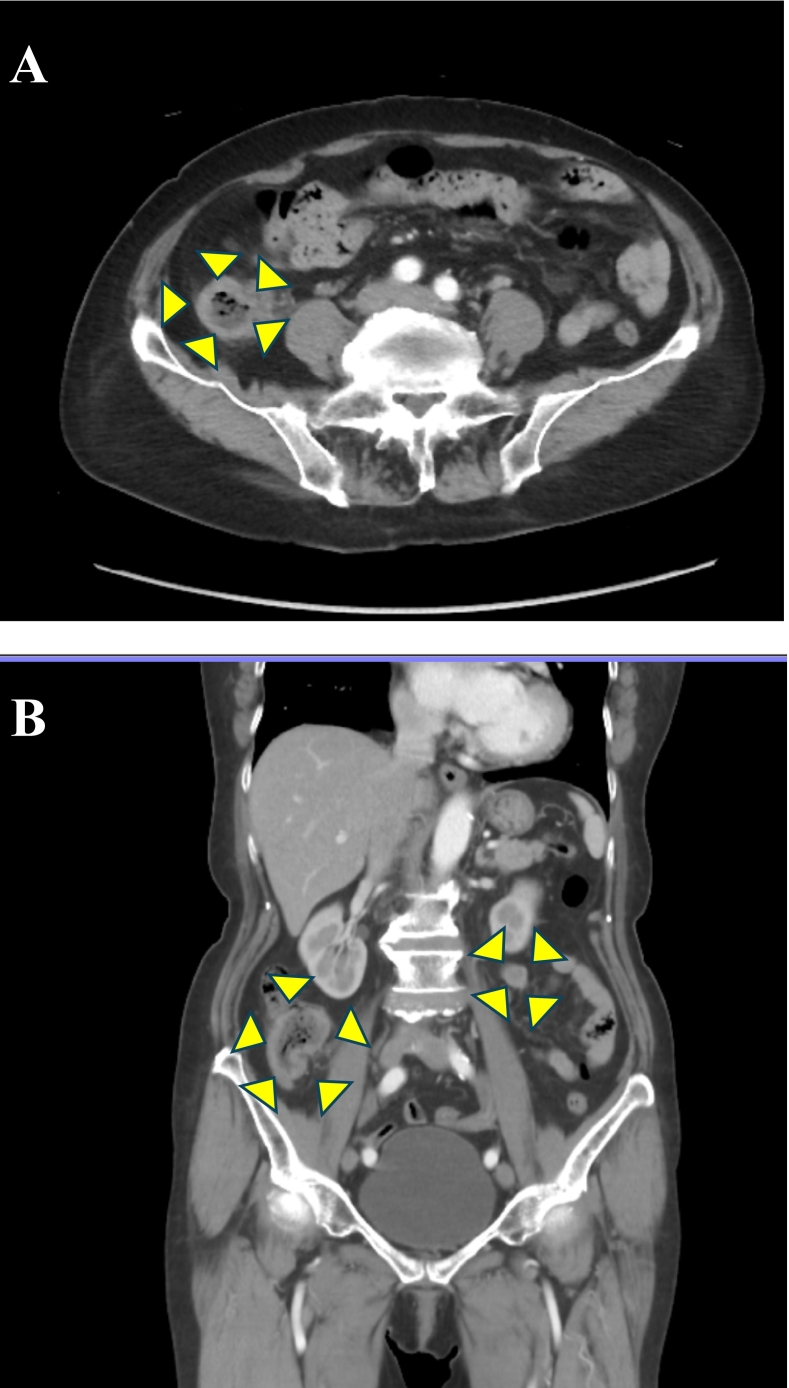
Abdominal computed tomography (CT). A and B: CT demonstrated an irregular wall thickening of 25 mm in the ascending colon and a 37 mm tumor in the mesentery of the small intestine, but no other obvious lesions.
